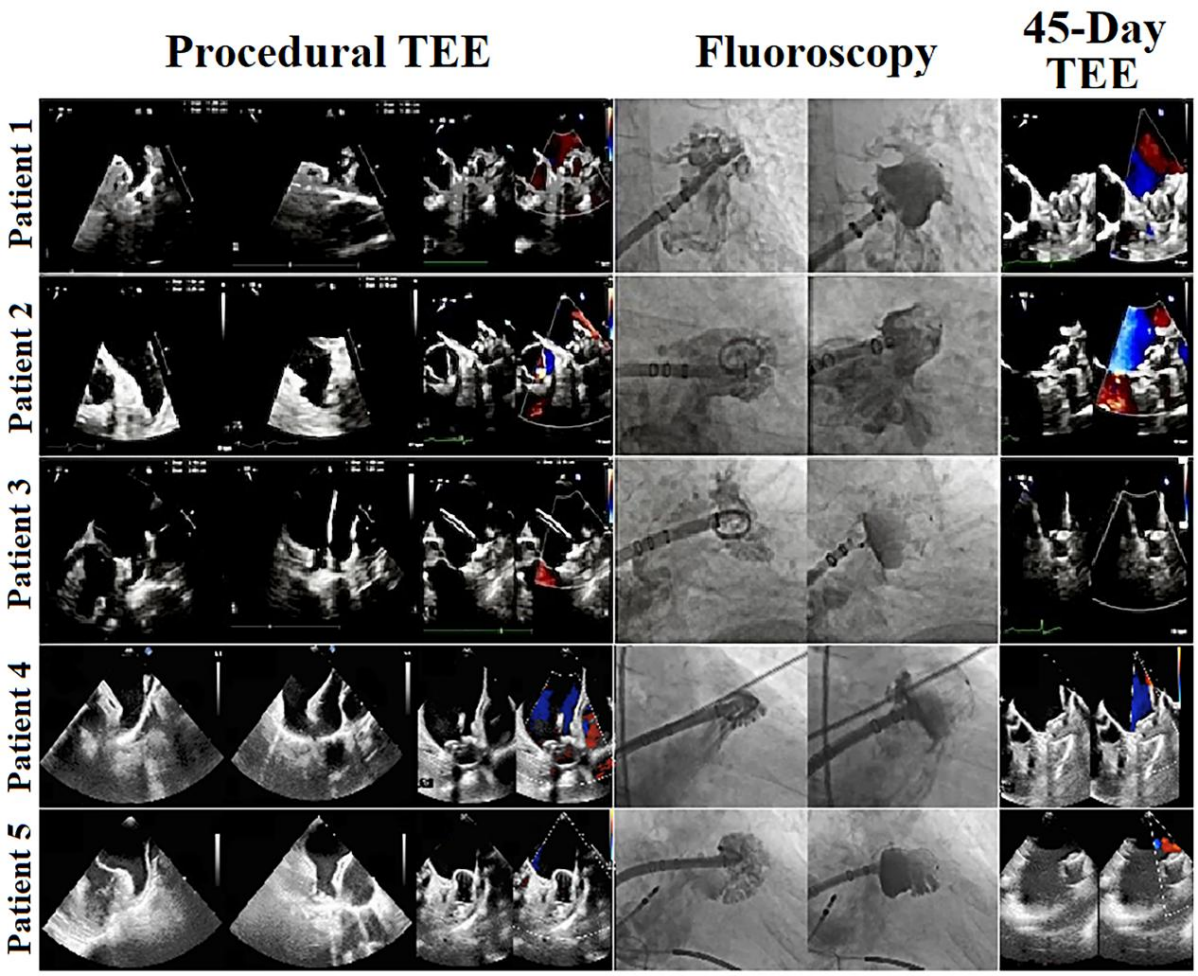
Successful implantations of WATCHMAN FLX devices using the SOLO-CLOSE strategy with 45-day postoperative transoesophageal echocardiography revealing complete sealing with no peri-valvular leaks. TEE, transoesophageal echocardiography.
